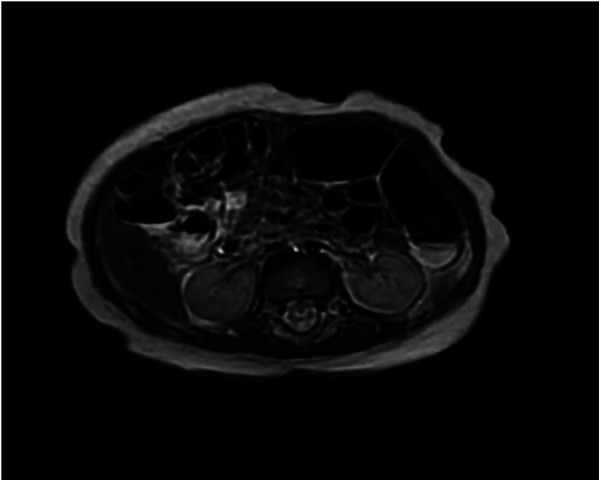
MRI images of the kidney (seven weeks post-discharge). T2-weighted fat-suppressed sequences within the left kidney. Both kidneys maintained normal dimensions, with the right kidney exhibiting no abnormal signal intensities.
